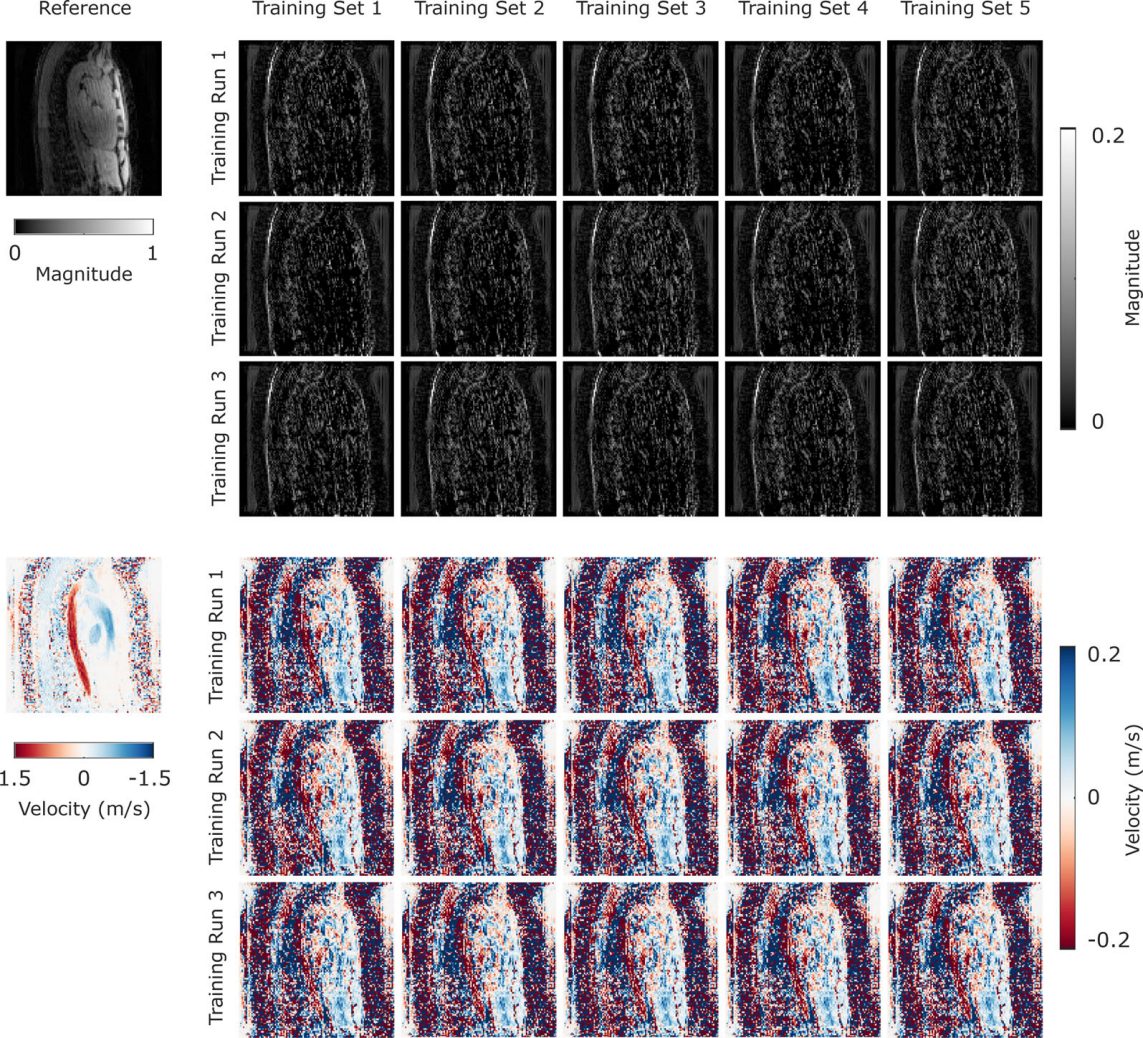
Examples of 4D Flow MRI reference magnitude and velocity images (first column) for data from Site A. For each row, columns 2–6 show the differences between the reference and images reconstructed with models trained on 1–5 (Training Set 1–5 respectively) datasets. Note that there are no apparent systematic differences due to the size of the training set.
